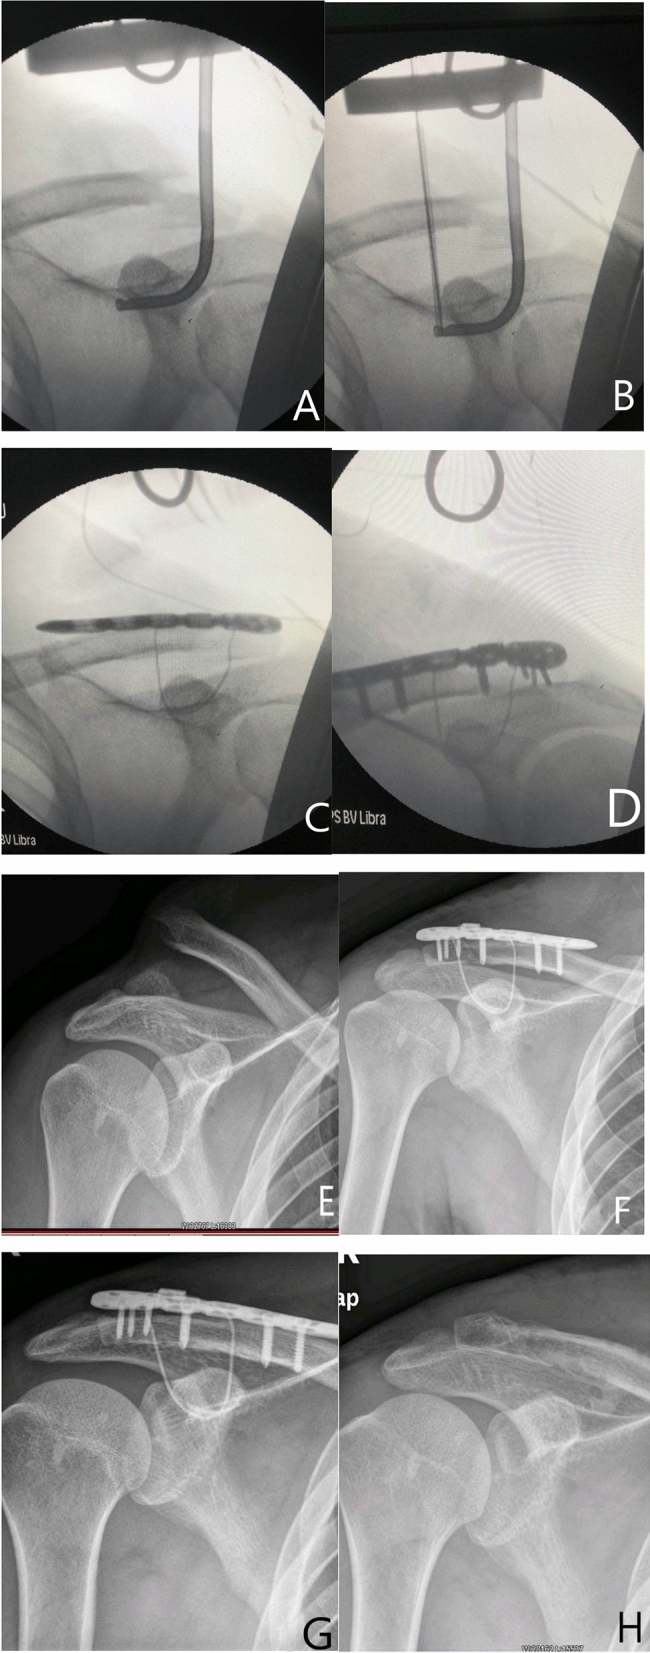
(A,B) The guide was inserted through the tunnel. (C,D) A titanium cable was inserted and the clavicle distal locking plate was fix. (E–H) A 27 years old man with right distal clavicle fracture. The distal fragment more than 2.5 cm, a titanium cable was inserted and the clavicle distal locking plate was fix. (E) Preoperative X-ray of the shoulder; (F) postoperative X-ray, (G) 3 months after operate and fracture healing, (H) the final follow-up 12 months after surgery and the implant removed.
